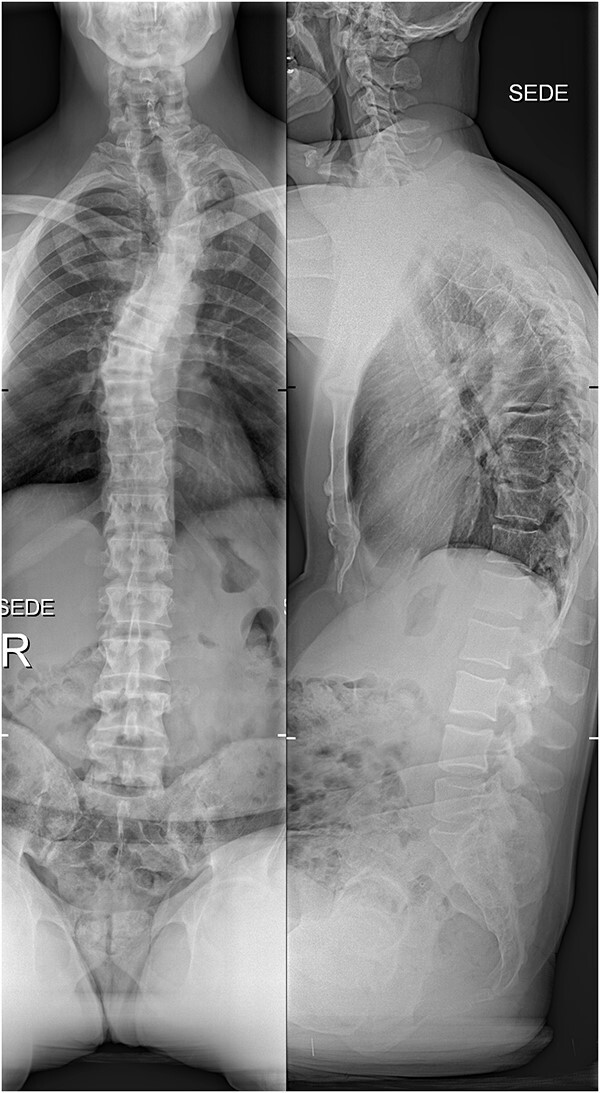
The initial X-ray images of the thoracic spine at the time of admission to our institution. Anteroposterior view (left) and lateral view (right).
